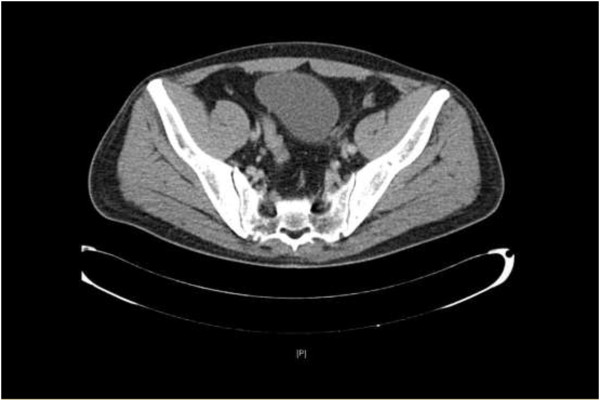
Computed tomography showed shrunken lesions in left inguinal region, and near-bilateral iliac vessels in the ninth postoperative month.
